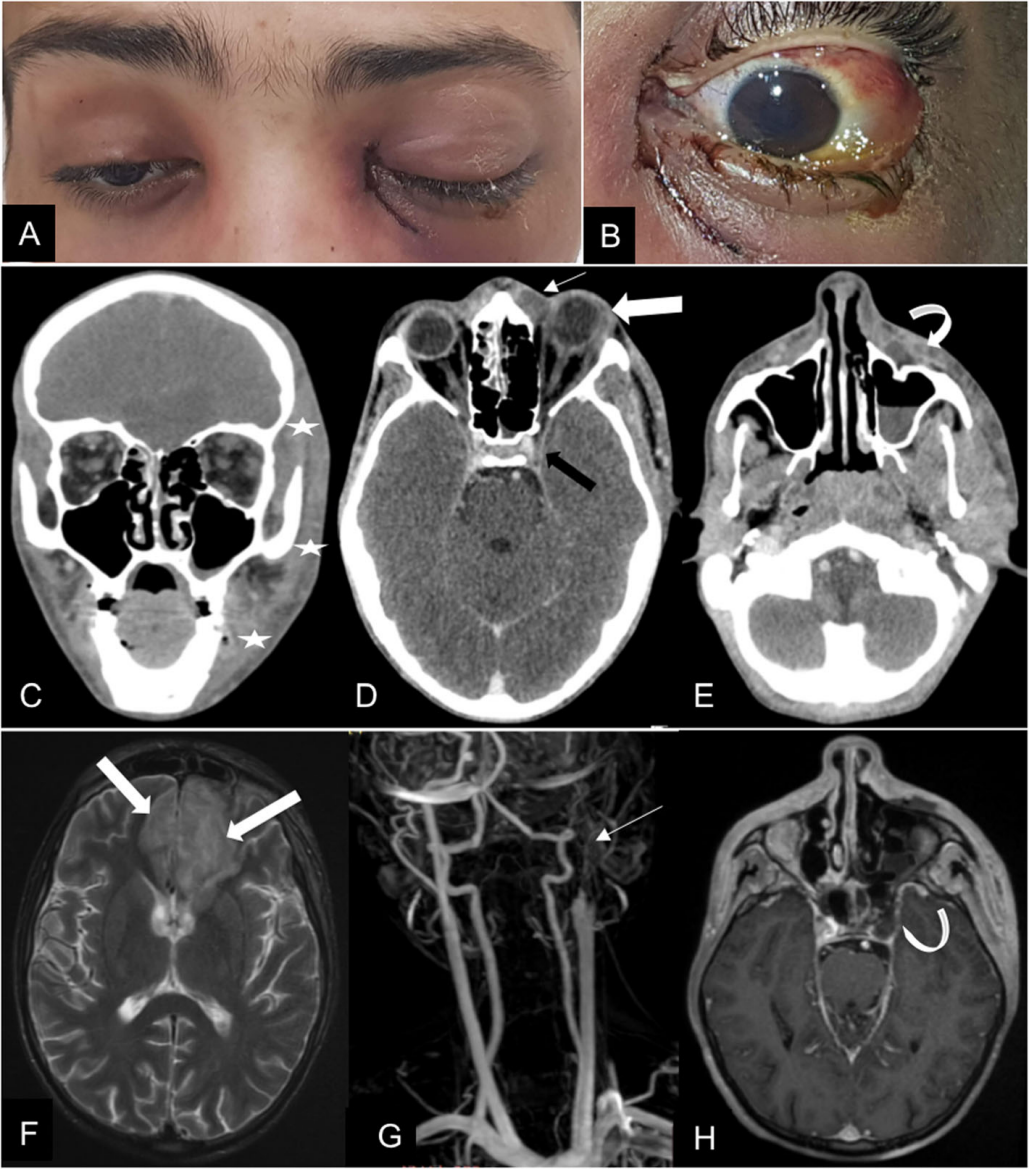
A 20-year old COVID-19-affected patient presented with rhino-orbitocerebral mucormycosis. (A) Complete blepharoptosis and inflammatory signs are seen on the left side. There is a mild proptosis on the right side. (B) Note the conjunctival injection, the chemosis and the corneal oedema. (C) Coronal and (D, E) Axial contrast-enhanced brain CT scan show thickening with infiltration of left hemiface fat planes (white stars); left pre-septal collection (arrow) with exophthalmos and lengthening of the antero-posterior axis of the eyeball (large arrow). This collection reaches the cellulo-fatty tissues next to the left maxillary sinus (curved arrow), the latter is the site of a partial liquid filling. The left cavernous sinus shows signs of thrombosis (black arrow). (F) axial T2 weighted image MRI showing T2 hypersignals (large arrow) in areas of the frontal lobes confirming endocranial extension. (G) MR angiography shows left carotid artery thrombosis (arrow). (H) 3D T1 weighted sequence with contrast shows left cavernous sinus thrombosis (curved arrow)
